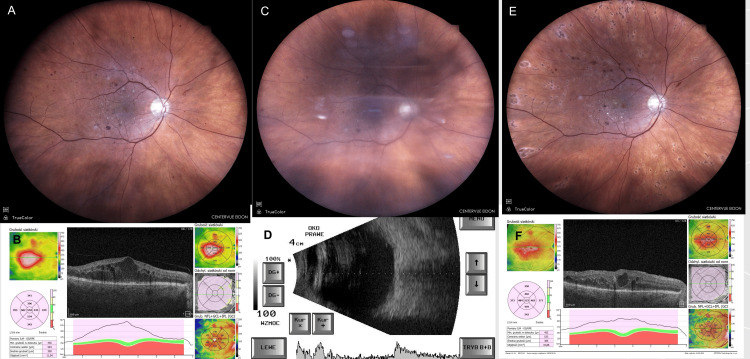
Case 1: patient treated for DME who developed endophthalmitis after the sixth bevacizumab injection. (A) Before inflammation: DME with maculopathy, fundus photo (taken on the day of injection). (B) Before inflammation: DME, OCT image (taken on the day of injection). (C) Sterile endophthalmitis: retina barely visible, fundus photo (taken 24 hours after injection). (D) Sterile endophthalmitis: B-scan ultrasound (taken 24 hours after injection). (E) One month after CEVE: fundus photo (taken 30 days after CEVE). (F) Two months after CEVE: residual DME on OCT (taken 60 days after CEVE). Images taken at OCHO Nowy Sącz. Fundus photographs: iCare EIDON FA, iCare, Vantaa, Finland. OCT: Revo NX80 SOCT, Optopol Technology, Zawiercie, Poland. USG: Echoson, Echo-Son S.A., Warsaw, Poland. The OCT image during inflammation (corresponding to Figure 1C) could not be obtained due to vitreous inflammatory opacities; therefore, a B-scan ultrasound was included instead. Timeline: injection (August 21, 2024) → inflammation (August 22, 2024) → CEVE (August 22, 2024) → postoperative follow-up visits (seven and 30 days postoperatively). CEVE, complete and early vitrectomy for endophthalmitis; DME, diabetic macular edema; OCT, optical coherence tomography; RE, right eye
